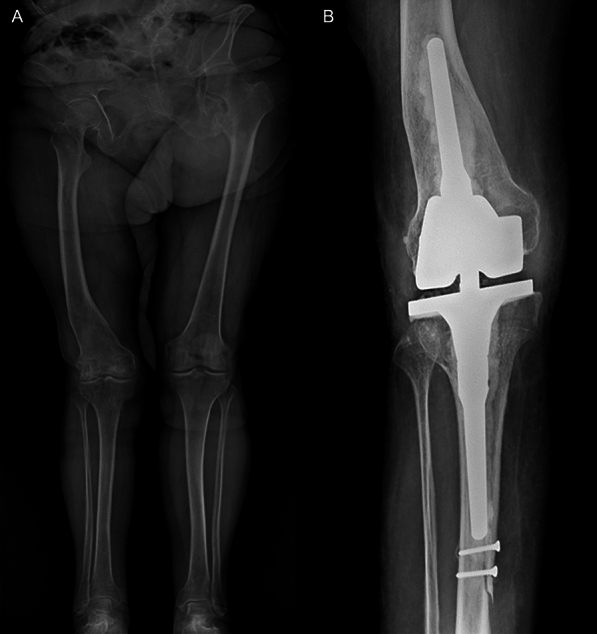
Case B.L., 77-year-old female; A The preoperative full weight-bearing X-ray shows right knee osteoarthritis with a femoral diaphysis deformity. B 1-year postoperative anteroposterior right knee X-ray shows the good positioning of the implant. During the reaming of the tibial diaphyseal canal, very narrow, an incomplete tibial shaft fracture was reported and synthesized with two mono-cortical screws
